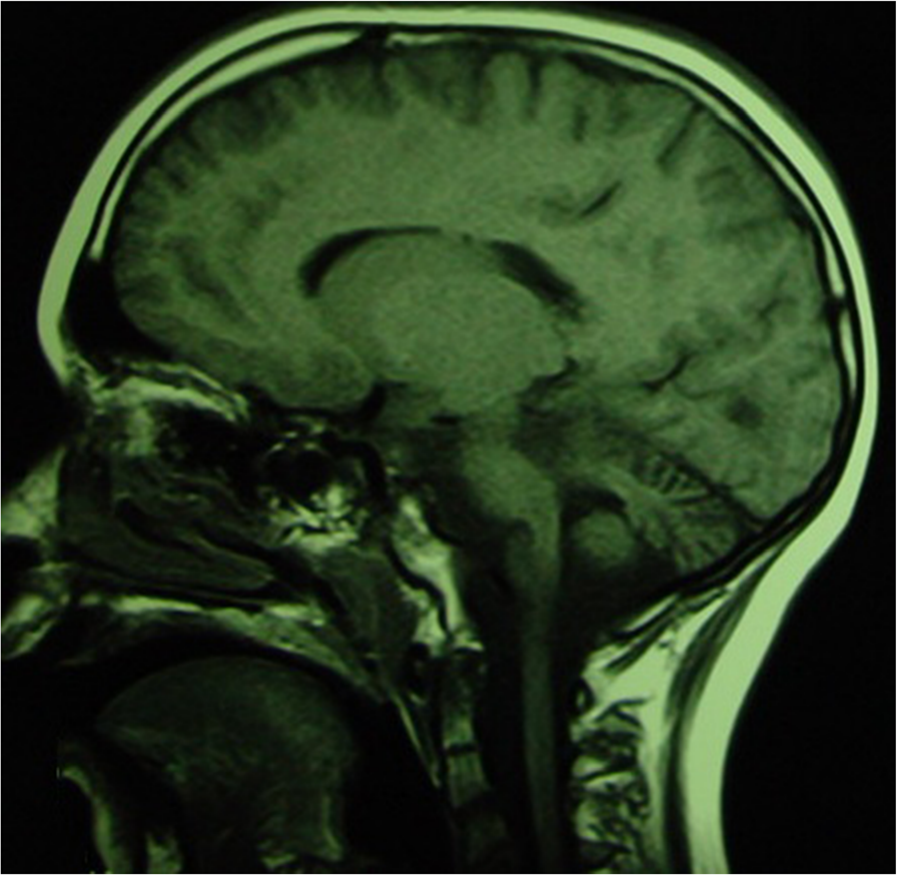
Sagittal T1-weighted MRI images of a 3-year-old aged patient presenting ponto-cerebellar dysplasia
